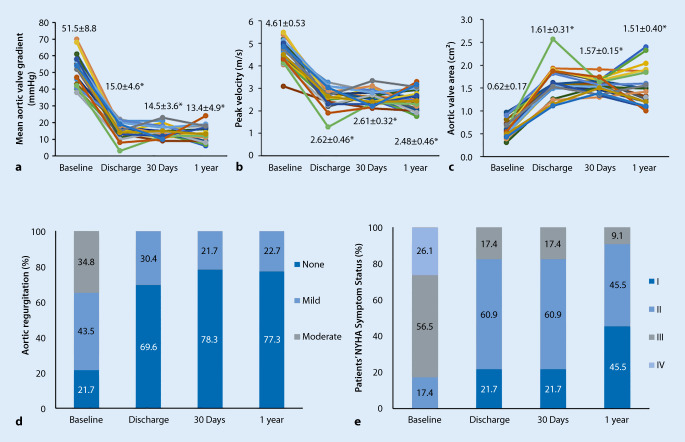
Valve hemodynamics assessed by transthoracic echocardiography and New York Heart Association (*NYHA*) functional class status. a Mean aortic valve gradient. b Peak velocity. c Aortic valve area. d Aortic regurgitation. e NYHA functional class status. ^*^*p* < 0.001 vs. baseline
